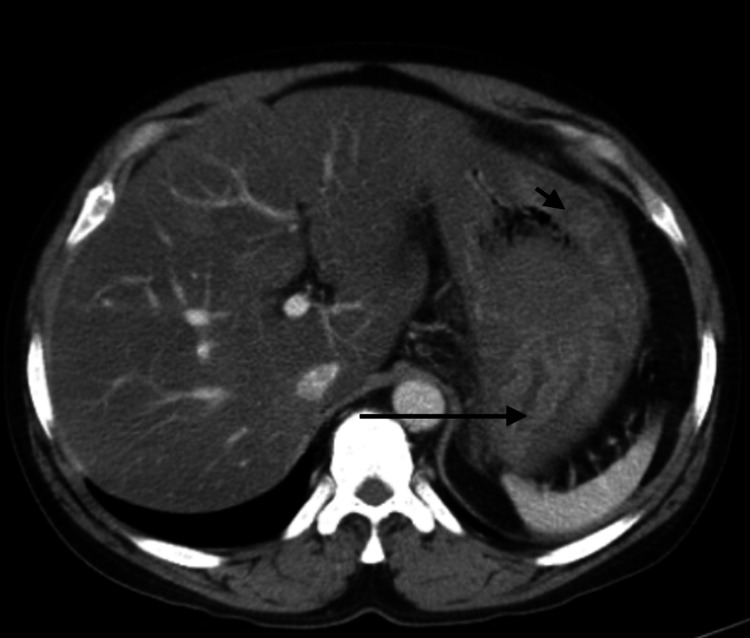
Computed tomography of the abdomen with contrast showing increased gastric wall thickness (arrowhead) and prominent gastric folds (arrow)
